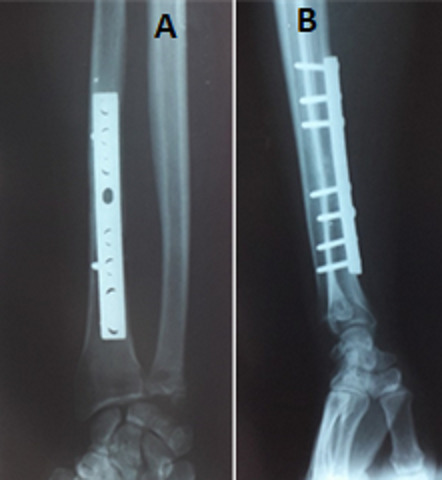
Radiographie des deux os de l'avant-bras prenant le poignet montrant la consolidation de la fracture (A) face; (B) profil
